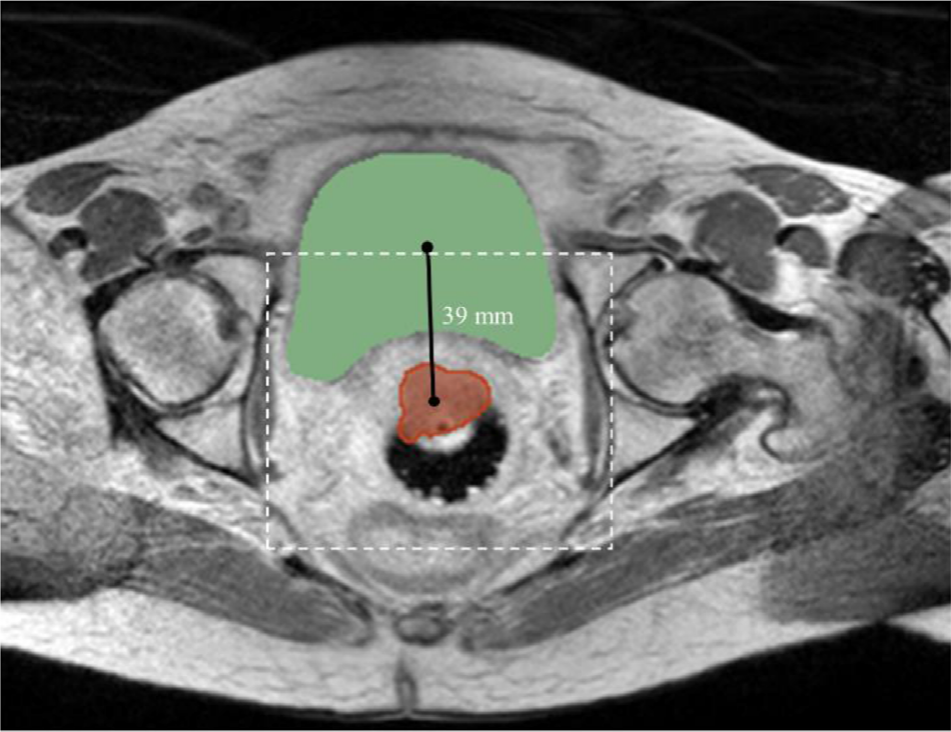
An example showing the bladder and clinical target volume (CTV) locational relationship along with the cropped region of interest (ROI) (white‐dashed window) in a typical female pelvic axial magnetic resonance imaging (MRI) in our dataset
